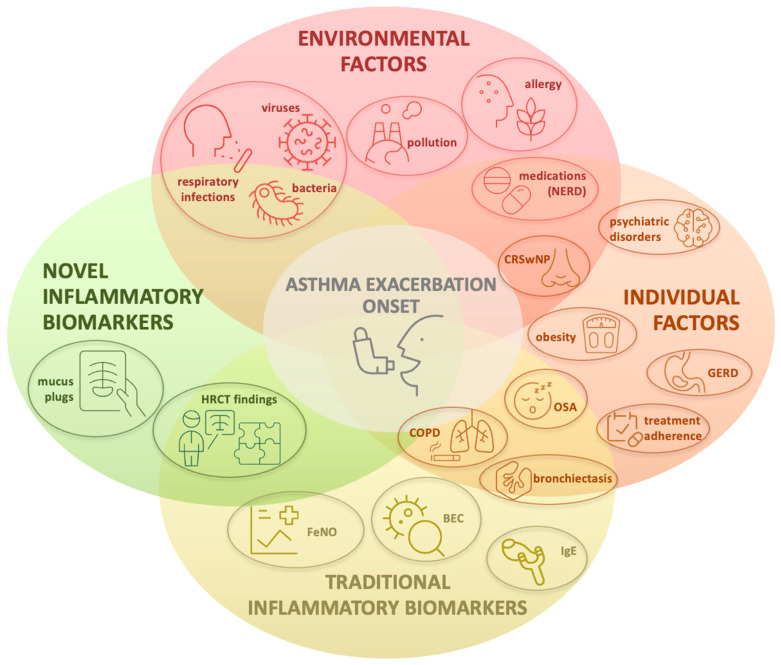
Mechanisms of asthma exacerbation’s onset. NERD: nonsteroidal anti-inflammatory drug-exacerbated respiratory disease; CRSwNP: chronic rhinosinusitis with nasal polyps; OSA: obstructive sleep apnea; GERD: gastroesophageal reflux disease; COPD: chronic obstructive pulmonary disease; FeNO: fractional exhaled nitric oxide; BEC: blood eosinophil count; IgE: immunoglobulin E; HRCT: high-resolution computed tomography.
